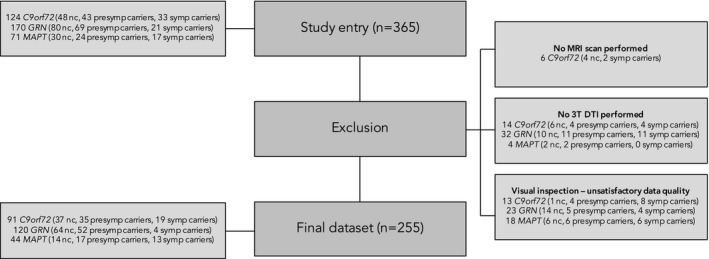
Overview of participant in‐ and exclusion. A total of 365 subjects were eligible for study participation. Six subjects did not undergo MRI scanning, and were therefore excluded. Only 3T scans were considered; therefore, 1.5T (*n* = 34) scans were first excluded from further analysis. Visual quality control of the images resulted in exclusion of another 54 images, leading to a final dataset of 255 subjects (115 noncarriers (“nc”), 104 presymptomatic mutation carriers, 36 symptomatic carriers.
